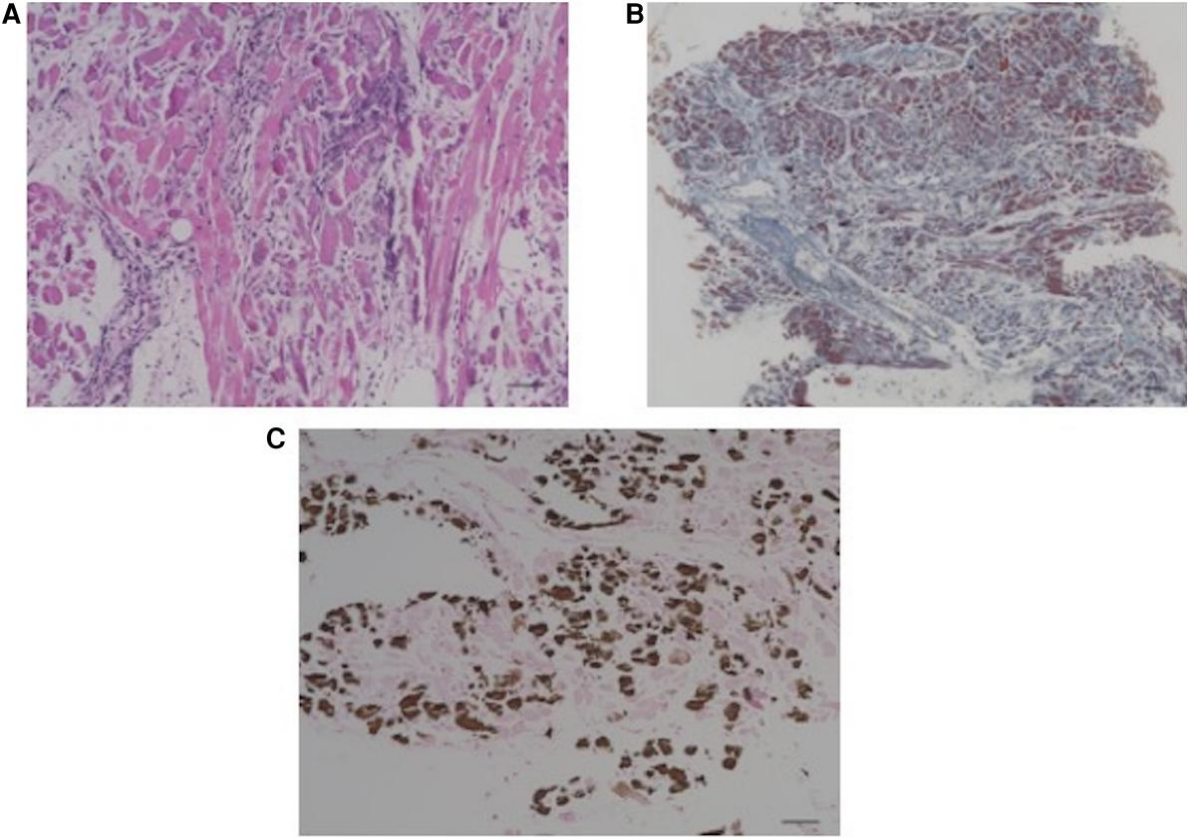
Case 2. A time course of myocardial calcification on CT (*A*) at admission and at (*B*) 2 months and (*C*) 5 months after admission. Biventricular myocardial calcification was detected at admission and became obvious during follow-up. Scale bars: 50 µm.
